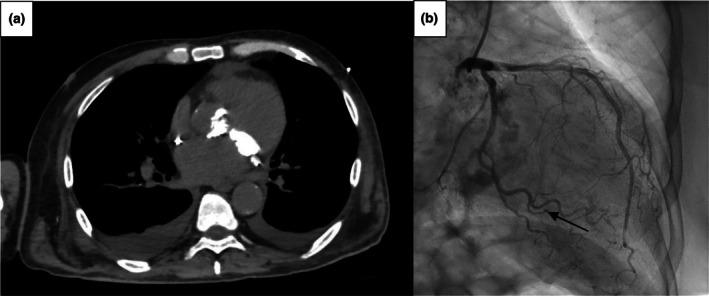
Valvular calcifications and MAC. CT scan showing a case of MAC in axial view, with diffuse calcifications of both the mitral and aortic valve; coronary angiography showing calcification of the mitral anulus (arrow). MAC, mitral annular calcification.
